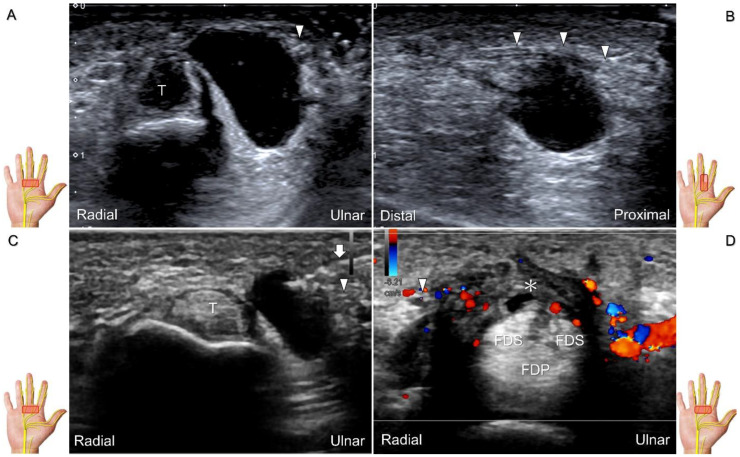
Ultrasound images demonstrate the entrapment of the palmar common digital nerve due to a ganglion seen in short-axis (A) and long-axis (B) views. Ultrasound-guided aspiration (C). Nerve entrapment due to annular ligament tear (asterisk) following an iatrogenic injury (D). Arrowheads: palmar common digital nerve; Arrow: needle; T: flexor tendon; FDS: flexor digitorum superficialis tendon; FDP: flexor digitorum profundus tendon.
